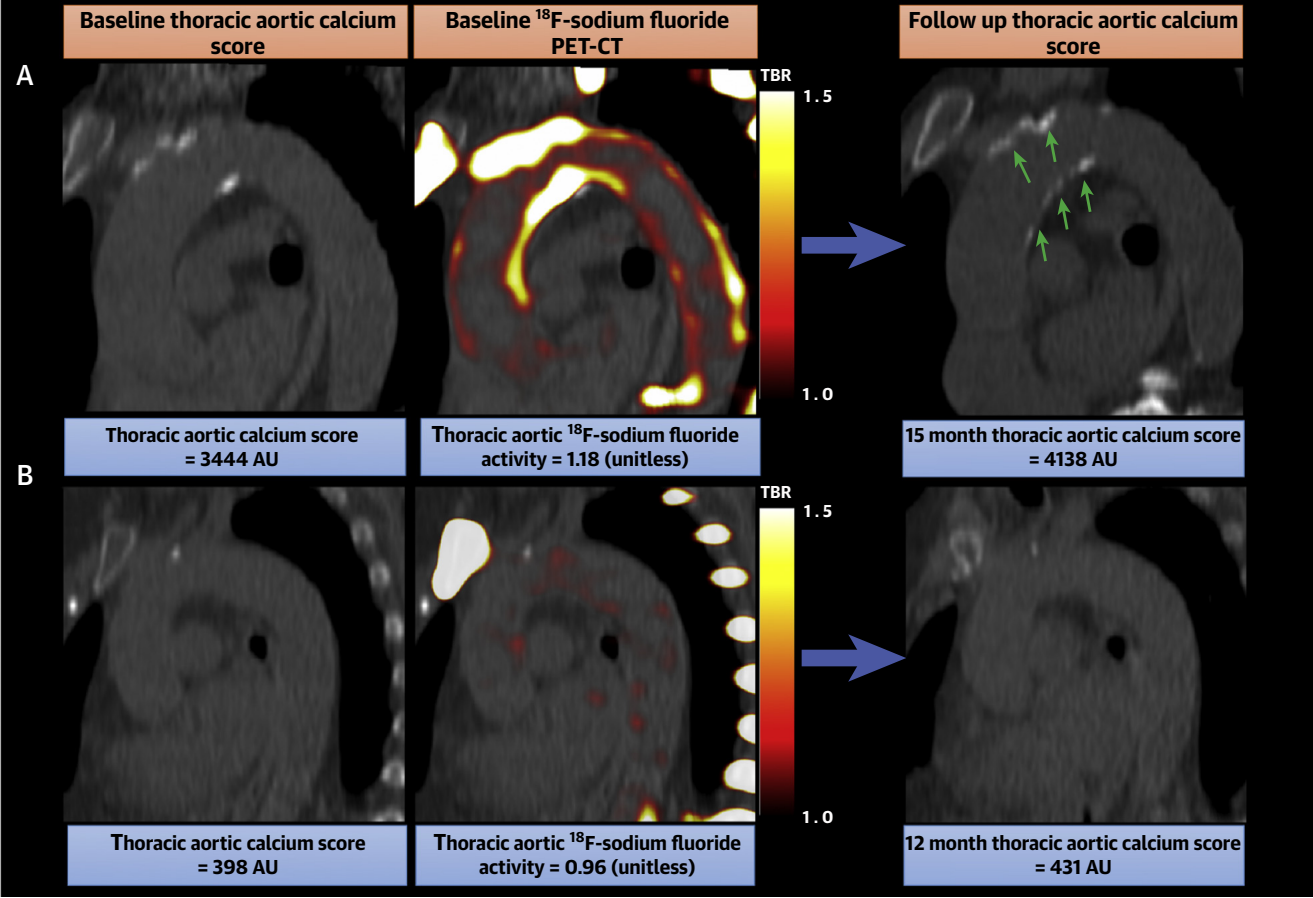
Thoracic Aortic ^18^F-Sodium Fluoride Activity and Progression of Aortic Calcification Relationship between thoracic aortic ^18^F-sodium fluoride activity and progression of thoracic aortic calcium score (Agatston units [AU]). (A) Example case in which intense ^18^F-sodium fluoride activity on positron emission tomography and computed tomography (PET-CT) precedes areas of macrocalcification on computed tomography. (B) Example case of low ^18^F-sodium fluoride activity and minimal progression of macrocalcification on computed tomography. TBR = tissue-to-background ratio.
